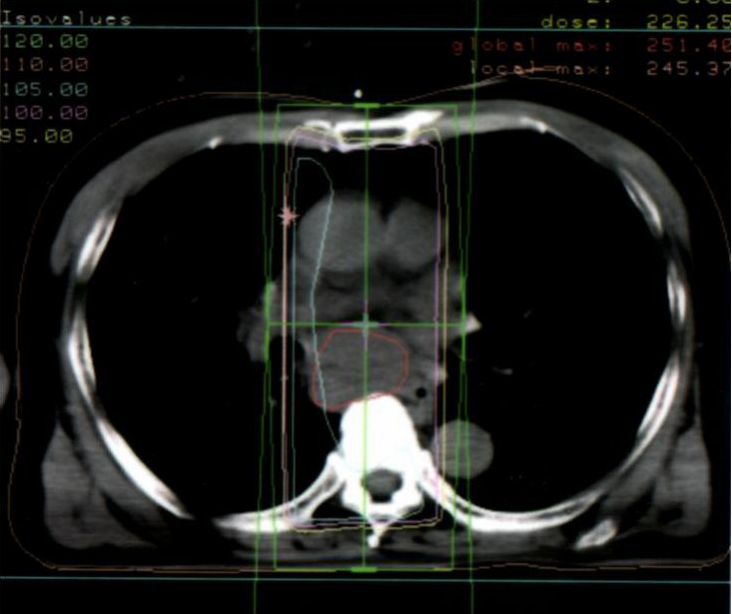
Chest CT image showing radiation dose distribution: axial view.
